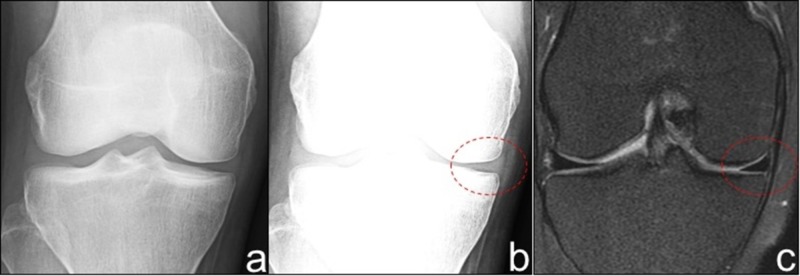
Radiographic appearance of a normally positioned meniscus. a) AP knee radiograph with standard window settings. b) AP knee radiograph with narrow window settings demonstrates visualized meniscal tissue without extrusion. c) Correlative mid coronal proton density fat saturation MRI confirms lack of meniscal extrusion. MRI - magnetic resonance imaging.
